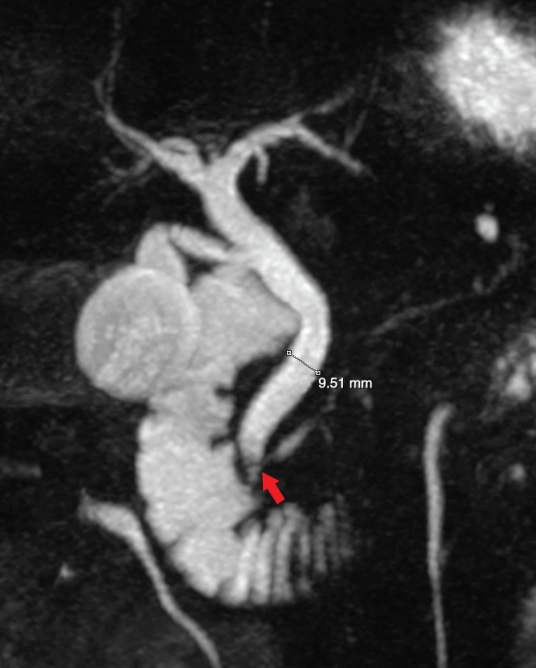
MRI *T*_2_ weighted maximum intensity projection three-dimensional reformat sequence depicting a newly dilated common bile duct ( >9 mm) and a small filling defect within the lower duct above the ampulla (red arrow).
